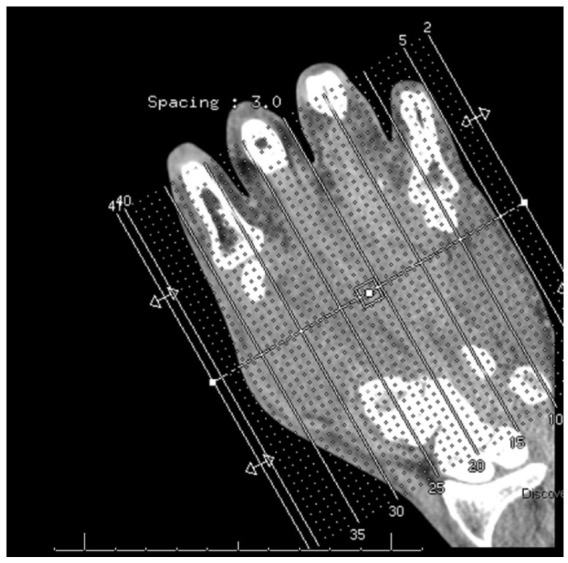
CT left-hand with contrast. No acute osseous abnormality of the left hand. Apparent diffuse prominence of the left thenar muscles, without soft tissue air or drainable fluid collection appreciated. CT: computed tomography.
